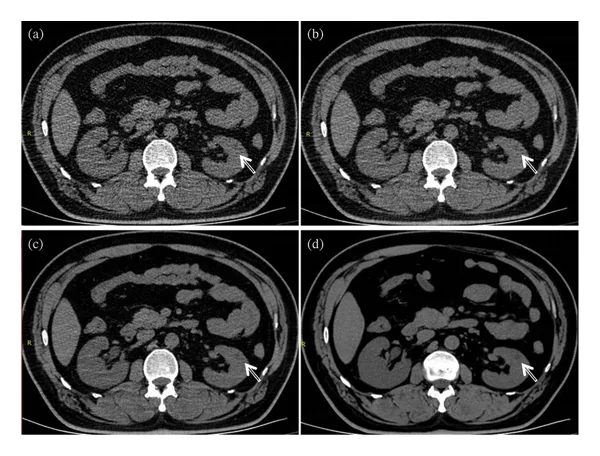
Comparative analysis of image quality differences among four reconstruction algorithms (FBP, iDose, PI Standard, and PI Smooth) in abdominal CT imaging. The images were derived from an 89‐year‐old male who underwent plain abdominal CT scanning, with corresponding reconstructed images using FBP (a), iDose (b), PI Standard (c), and PI Smooth (d); the subjective image quality scores of these images were 2, 3, 4, and 5, respectively. On the plain CT images, a cystic low‐density lesion is visible in the renal parenchyma of the left kidney.
